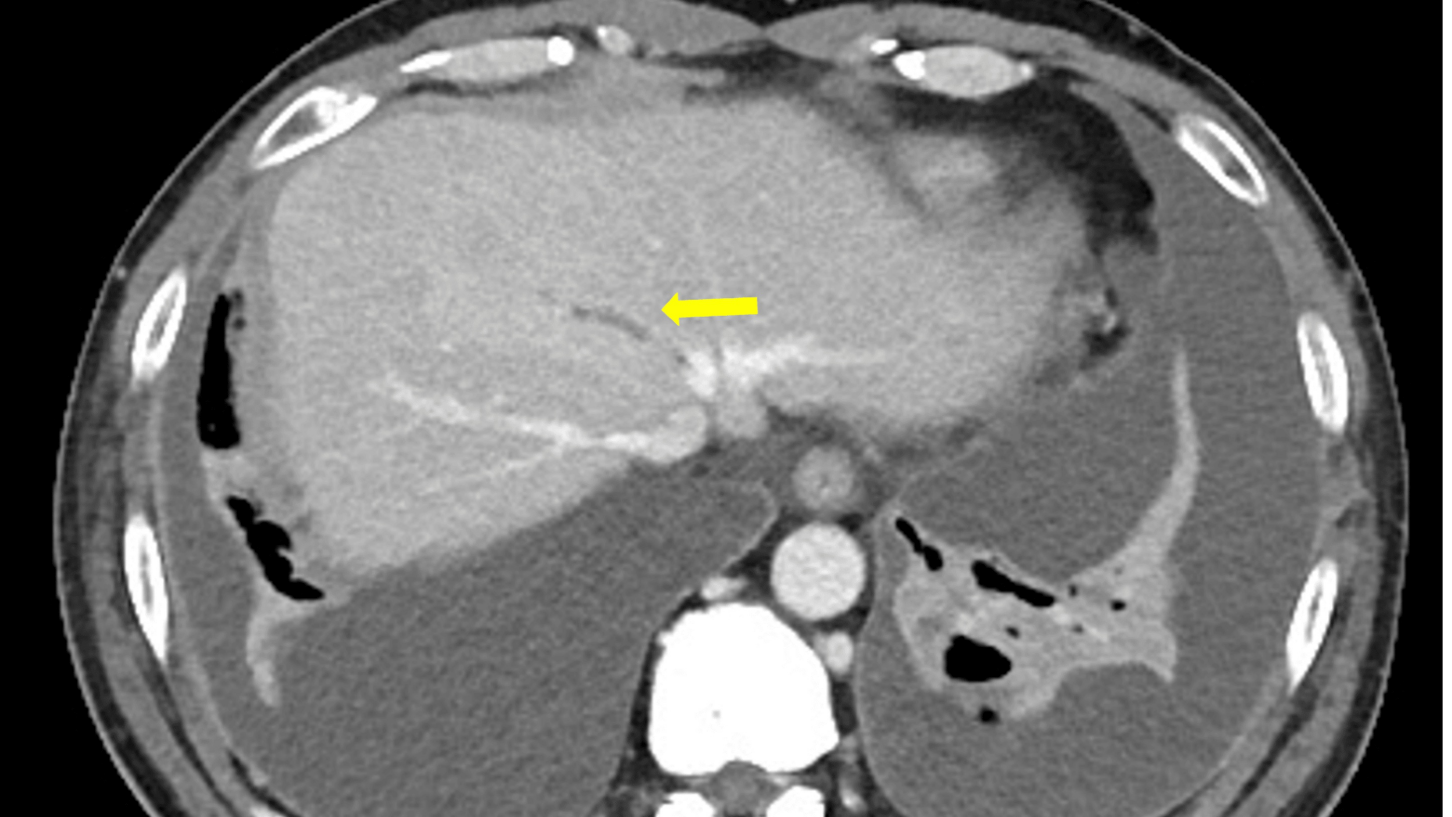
Contrast-enhanced abdominal computed tomography (CT) revealed hepatic vein thrombosis (yellow arrow).
